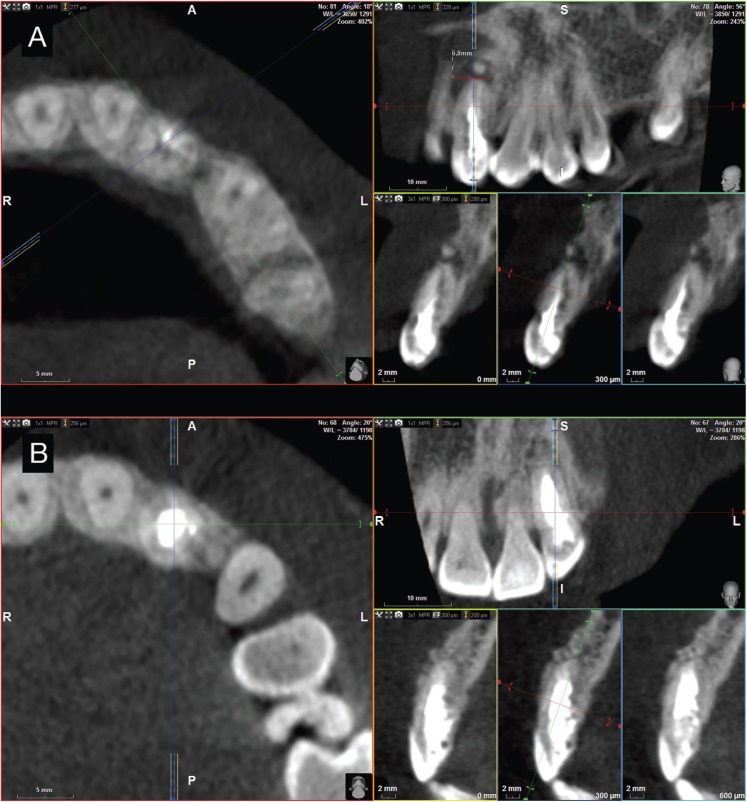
Preoperative cone-beam computed cross-sections. Notice apical fracture and gutta-percha presence (A). Cone-beam computed tomography cross-sections follow-up at 18 months after treatment. Complete periradicular healing (B).
